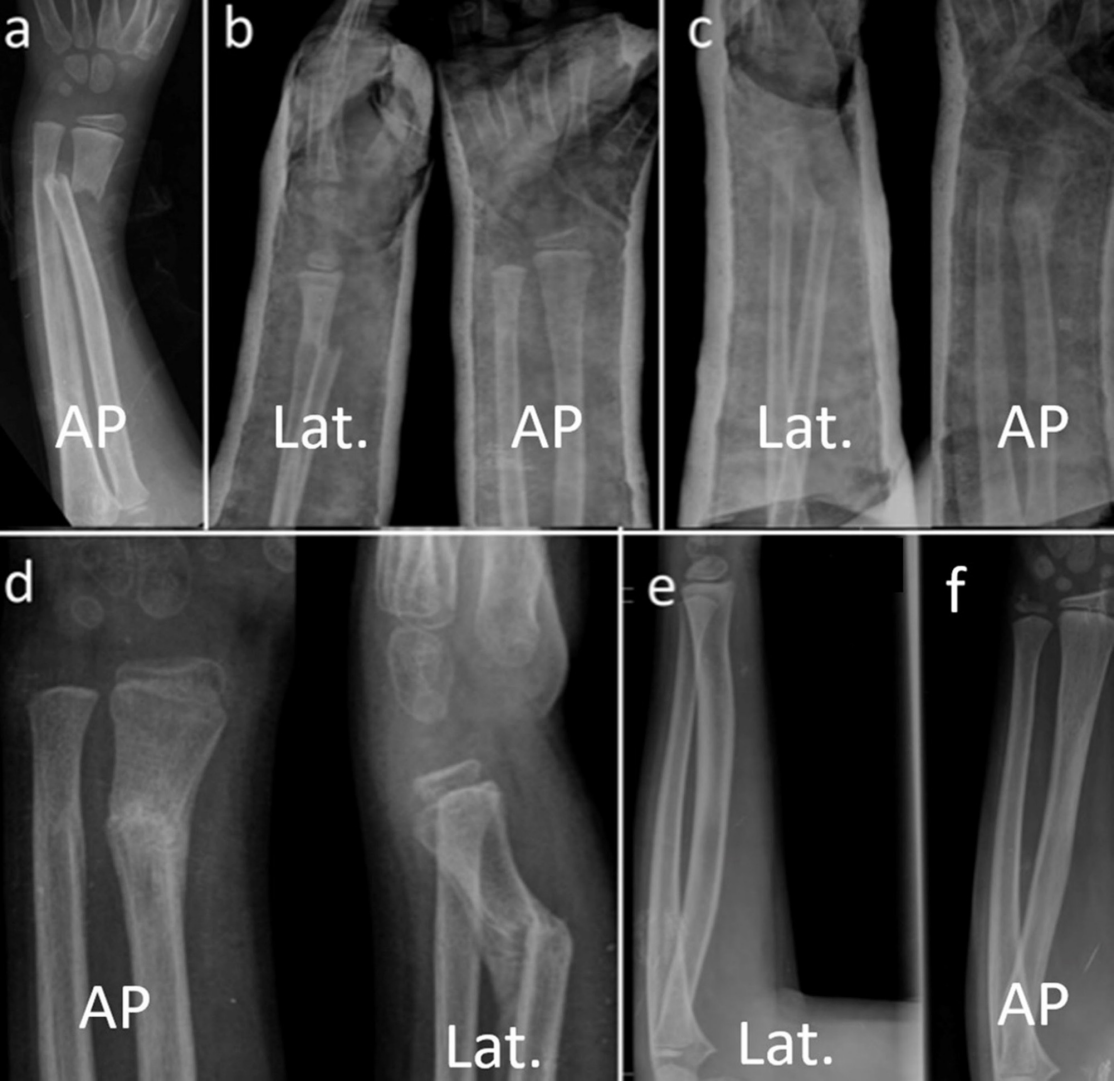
Case #2. Serial radiographic examination of eight-year-old girl with distal radial metaphyseal fracture. (a) Initial radiograph on admission. (b) Immediate closed reduction, the angulations were in acceptable range in both planes. (c) Re-displacement in cast. (d) Radiographs after the cast removal. (e,f) Final radiographs at 30th month showing full remodeling and normal alignment. AP: Anteroposterior; Lat.: Lateral.
